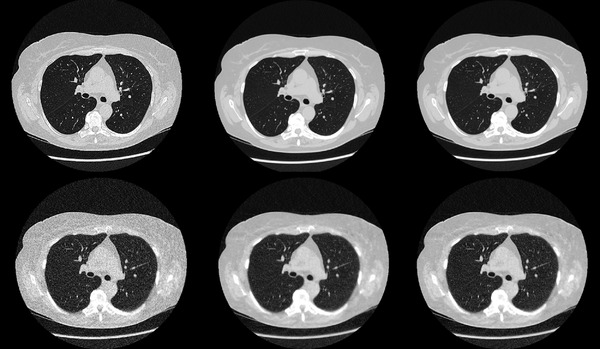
Axial CT slices obtained from the examinations performed using the full‐dose protocol (top row) and the ultra‐low dose (ULD) protocol (bottom row). The images are reconstructed using different reconstruction settings. Left: ASIR‐V 40%. Middle: DLIR‐H. Right: DLIR‐H + E2
